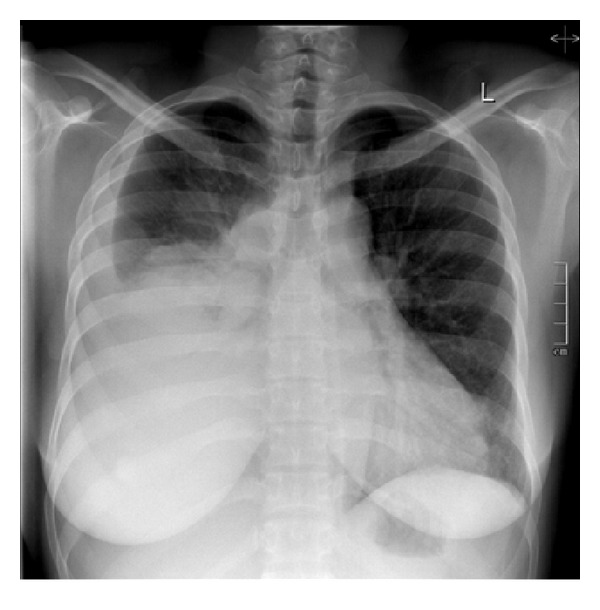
25-year-old female patient with right pleuroperitoneal leak following CAPD. Anteroposterior radiograph of the chest demonstrates large right pleural effusion with some underlying consolidation.
